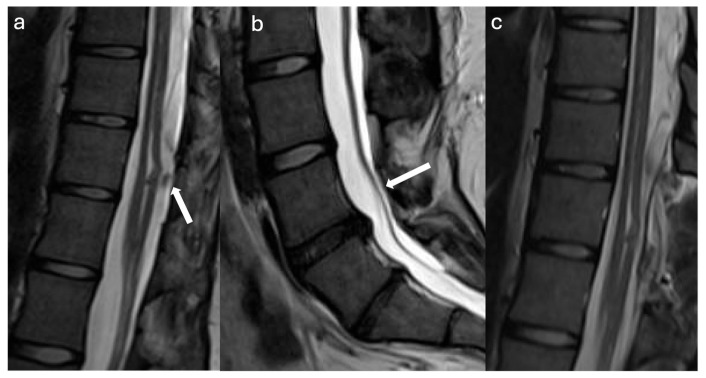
Radiographic Images Case 4: (a) Preoperative sagittal thoracic MRI T2 sequence shows a thoracic syrinx with maximal diameter at the T11 vertebral level. The cord shows a focal irregularity at that level most consistent with the syringosubarachnoid shunt exit site (arrow) suspicious for focal arachnoid tethering. (b) Preoperative sagittal lumbar MRI T2 sequence shows the previous laminectomy site (arrow) at L4–5 with a dorsal displacement of the cauda equina suspicious of cauda equina tethering most likely associated with a dural retethering of the residual filum terminale at the previous level of filum sectioning (arrow). (c) Early postoperative thoracic MRI T2 sequence shows only a slight reduction in syrinx diameter; however, an improvement of the spinal cord dorsal contour after exploration and removal of the subarachnoid shunt catheter is shown.
